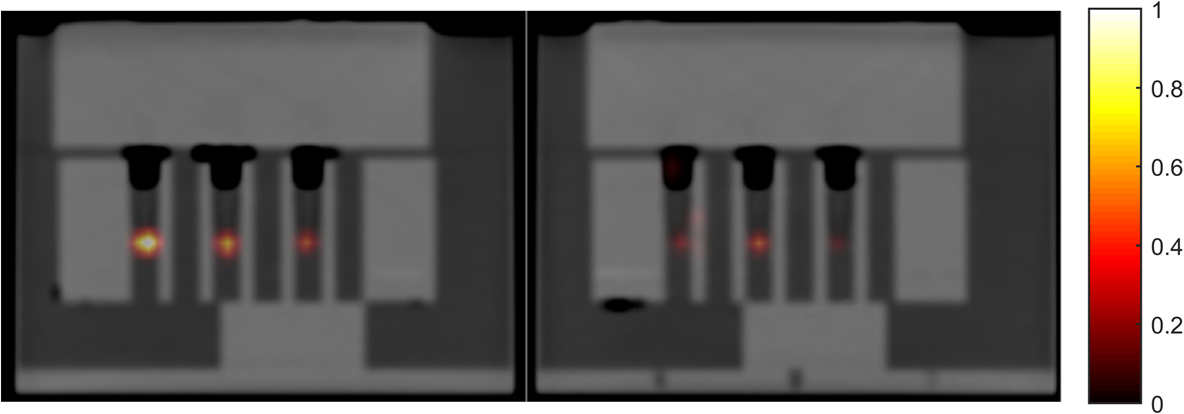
Ga68-PSMA-11-PET/CT image of different Eppendorf tubes all containing a pellet with 2 × 10^5^ HCT116+LNCaP cells. From left to right, the relative amount of LNCaP cells in the pellets is 100, 50, 25, 12.5, 5, and 2.5%, respectively
